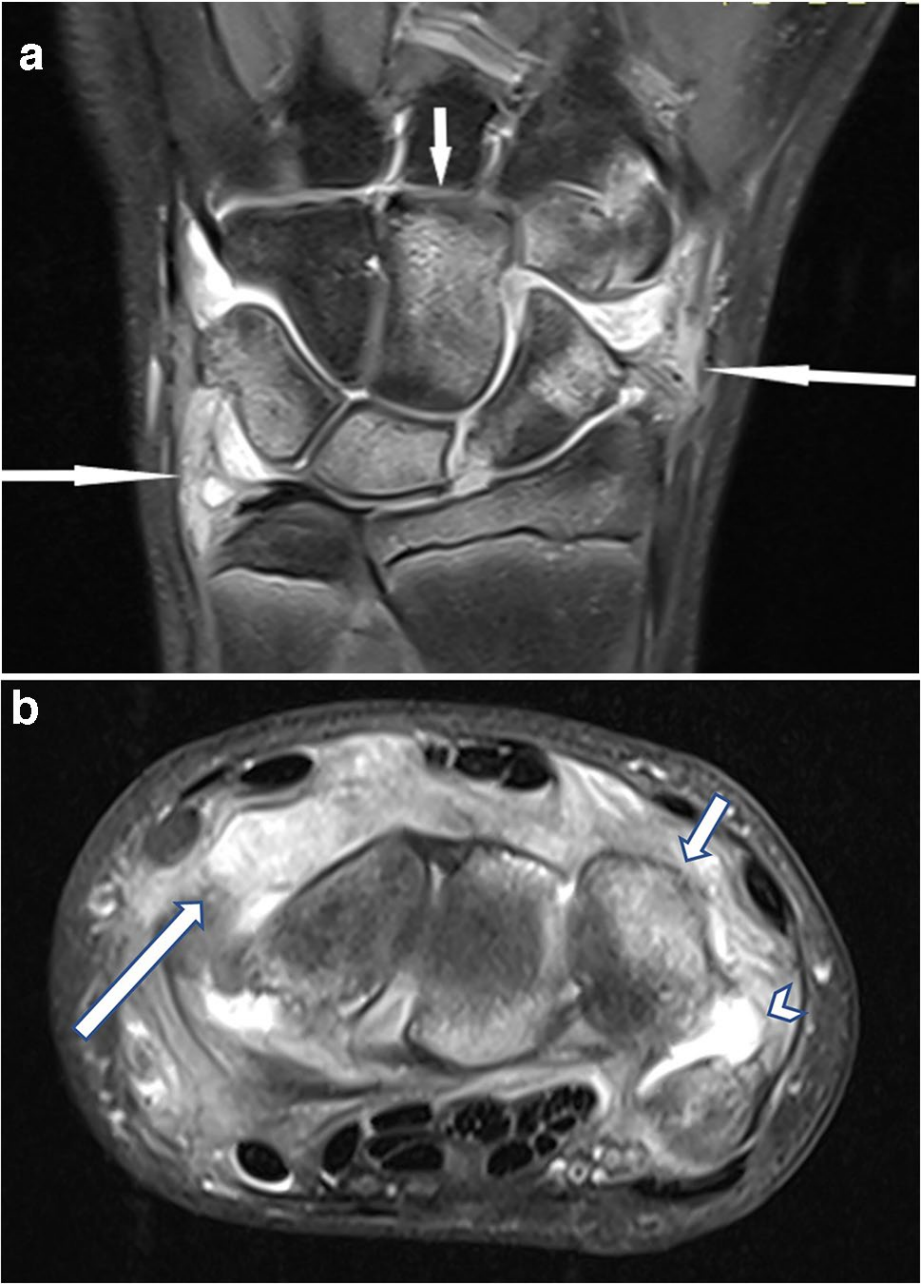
Magnetic resonance imaging of the right wrist in a 14-year-old boy with juvenile idiopathic arthritis with enthesitis (HLA B27 positive). Coronal (a) and axial (b) proton-density-weighted fat-suppressed images show marked synovial proliferation (*long arrows*), reactive edema of the carpal bones (*short arrows*), and a small joint effusion (*arrowhead* in b)—signs of synovitis
